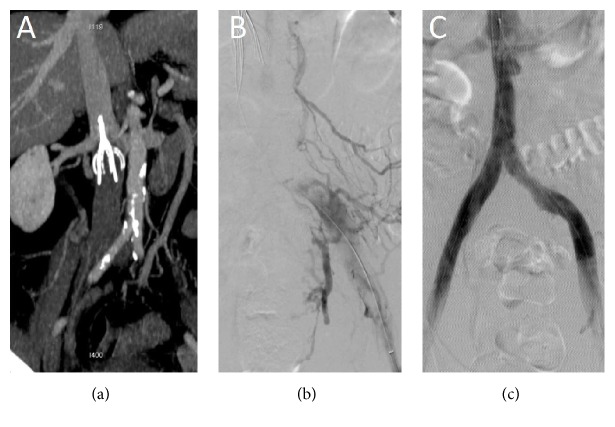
US-assisted CDT of IVC thrombosis using EKOS device. (a) Coronal reformatted contrast enhanced CT image demonstrates an IVC filter with thrombosis extending to the iliac veins. (b) Following puncture of the common femoral veins, a bilateral EKOS device was placed and 0.5 mg/hr tPA was infused for 8 hours from each groin. (c) Postvenogram demonstrates complete resolution of the thrombus with minimal thrombus at the apex of the IVC filter. This filter was subsequently removed.
